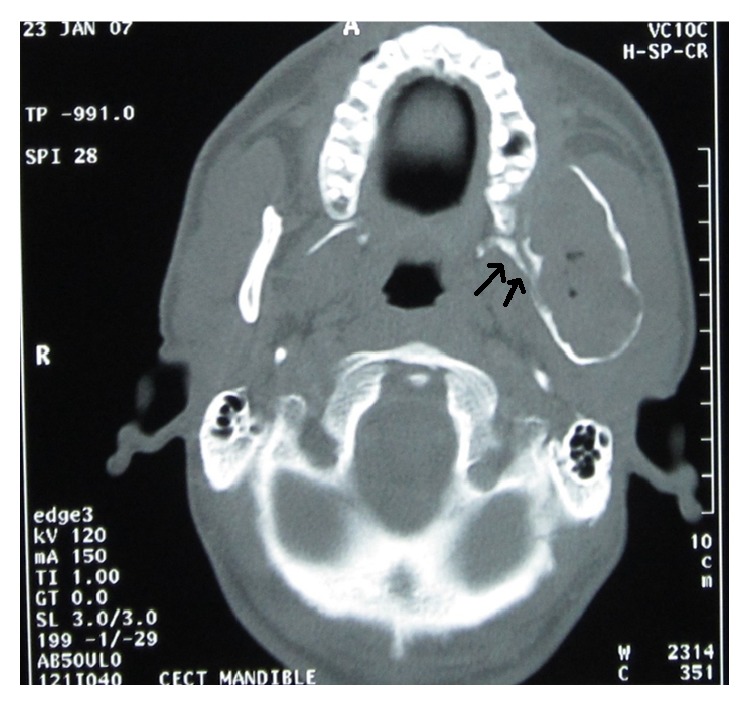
Transverse section of CT showing deflection of the left lateral pterygoid plate medially also with the left maxillary alveolar crest.
